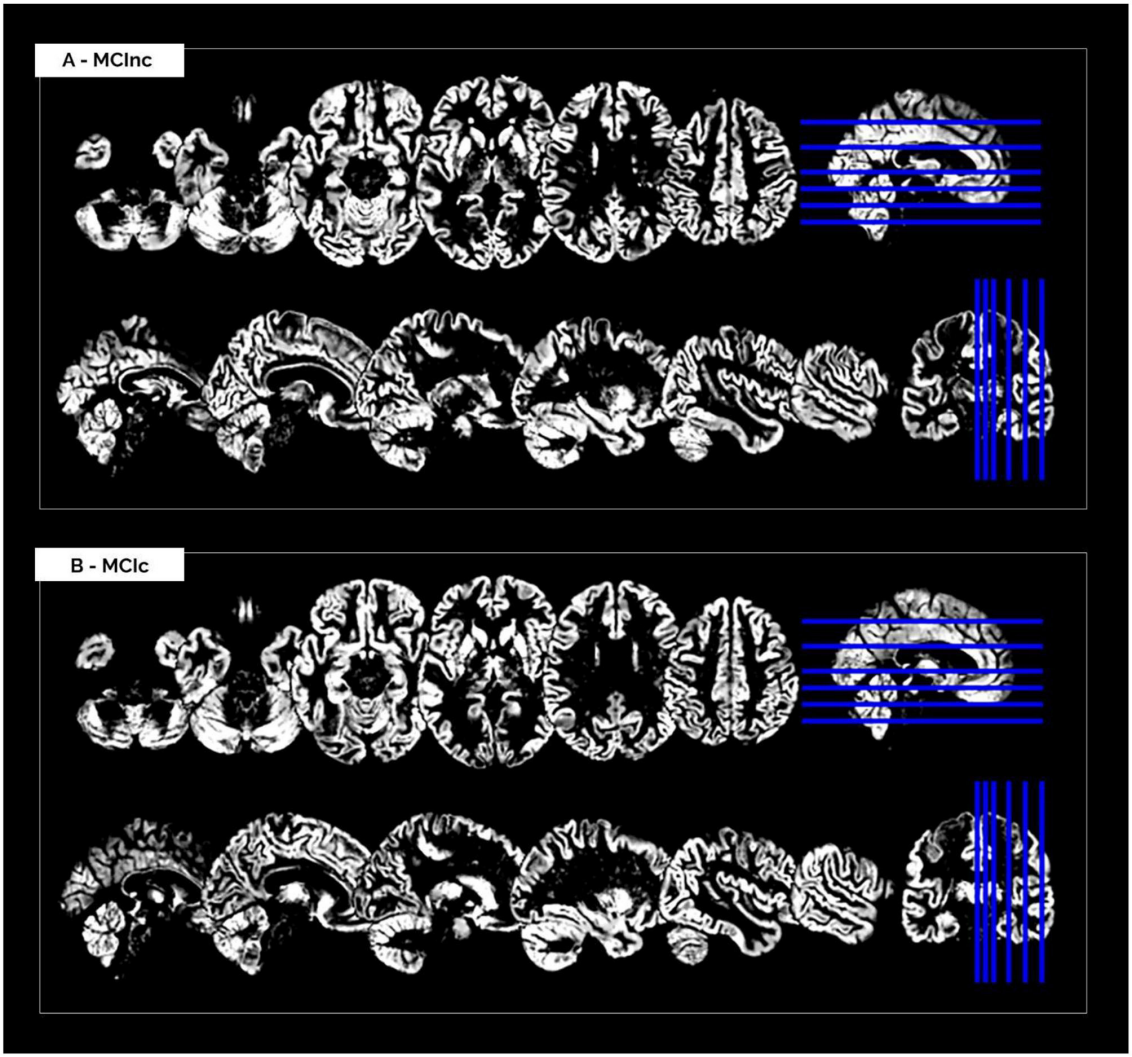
Cerebral MRI sections of an MCInc patient (A) and an MCIc patient (B) from the *Moradi-264* dataset after preprocessing (including co-registration to MNI template and segmentation into gray matter). Sections are shown in both axial (up) and sagittal (down) views. In the axial view, slices 20, 30, 40, 50, 65, and 80 are reported; in the sagittal view, slices 61, 66, 71, 80, 90, and 100 are reported.
